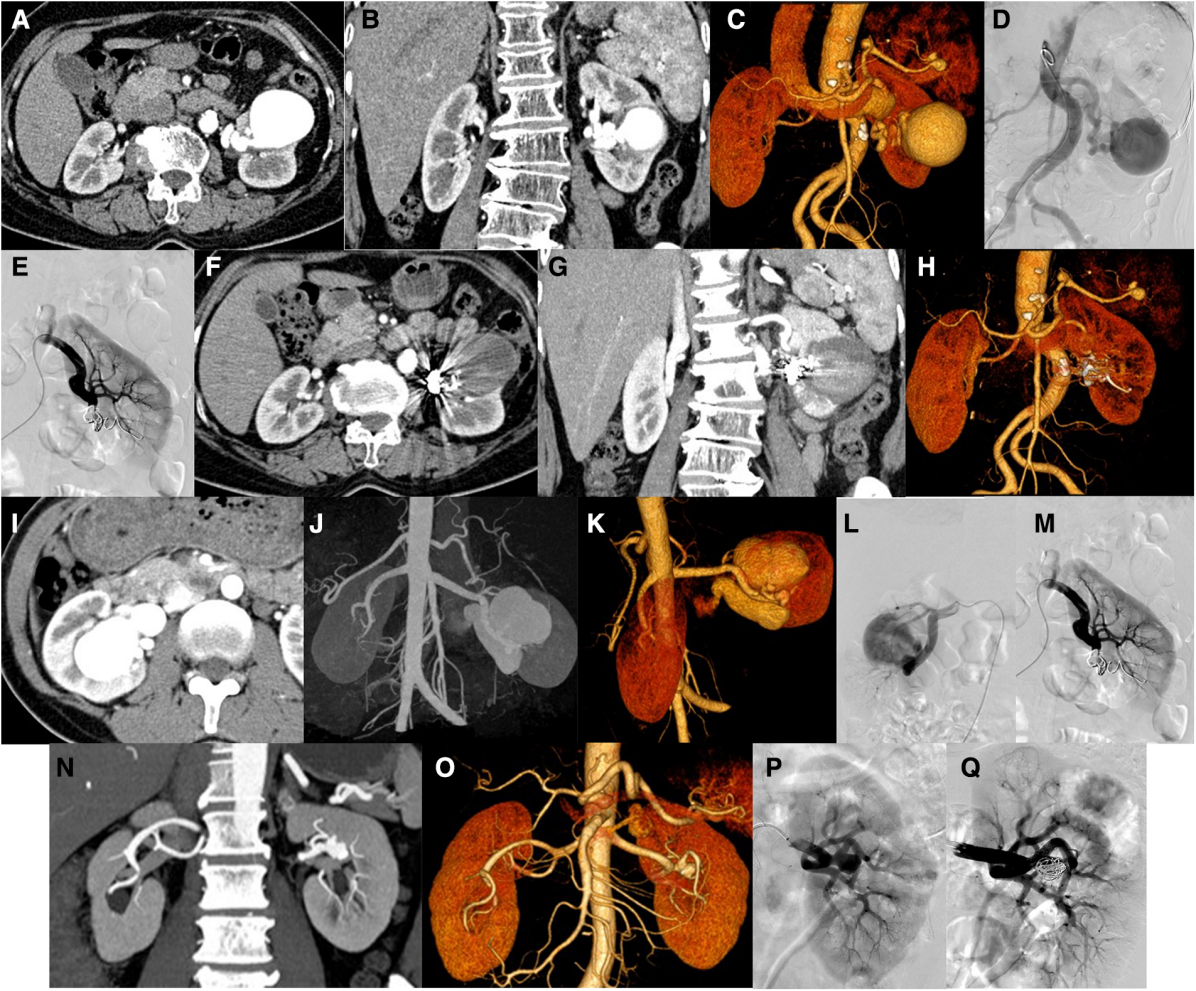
Patients no. 6 with renal aneurysm: (A,B) Computed tomography (CT) showed that the distal branch of the left renal artery (equivalent to the middle and lower part of the left kidney) showed a cystic dilation (approximately 4.9 cm × 4.5 cm × 4.9 cm), and multiple tumor-like dilations occurred in the proximal part of the splenic artery and the lumen near the splenic hilum. (C,D) An angiogram of the left renal artery showed that the left renal artery thickens, equivalent to a saccular dilated blood vessel (approximately 5.5 cm in diameter) in the lower-left kidney. Left renal aneurysm was considered. After embolization of the blood supply artery with a controllable coil, the above-mentioned saccular dilated blood vessels were not observed after repeated angiography. (F–H) Reexamination of the CT scan 1 week after the operation showed that the original distal branch (equivalent to the middle and lower part of the left kidney) showed cystic dilation, but no enhancement was observed. Patients no. 3 with renal aneurysm: (I–K) Enhanced CT showed enlargement of the right kidney and lesions in the area of the right kidney. Considering that the arteriovenous malformation may be accompanied by aneurysm-like expansion (maximum diameter approximately 4.7 cm), an arteriovenous fistula. (L,M) An angiogram of the right renal artery showed two large aneurysms in the middle and lower Poles of the right renal artery, whose sizes were 2.2 cm × 1.8 cm and 2.3 cm × 1.0 cm and they were in series. The responsible blood vessel of the right renal artery was embolized with microspring through the microcatheter, and Glubran was used for embolization. After embolization, the right renal aneurysm disappeared, and the right renal parenchyma was well displayed. Patients no. 13 with renal aneurysm: (N,O) Left renal aneurysm (maximum inner diameter of approximately 12.7 mm), and the left adrenal gland with a rich blood supply occupying the space, considering the possibility of pheochromocytoma or adenoma, bilateral repeated renal malformations. (P,Q) An angiogram of the left renal artery showed a wide-base aneurysm (approximately 11 mm in diameter) in the branch of the left renal artery and were placed at the base of the parent aneurysm with a stent. After embolization with a controllable coil, most of the aneurysm is blocked, the abdominal aorta is tortuous.
